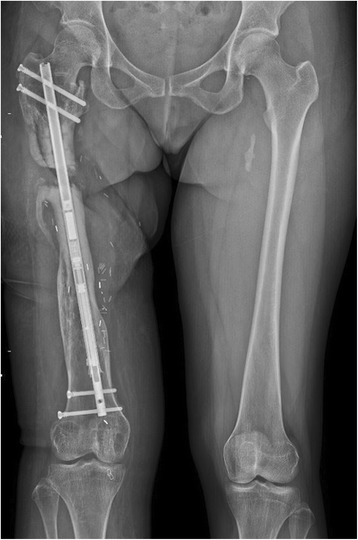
Long leg weight-bearing plain films demonstrating femur at ten weeks after restarting lengthening
